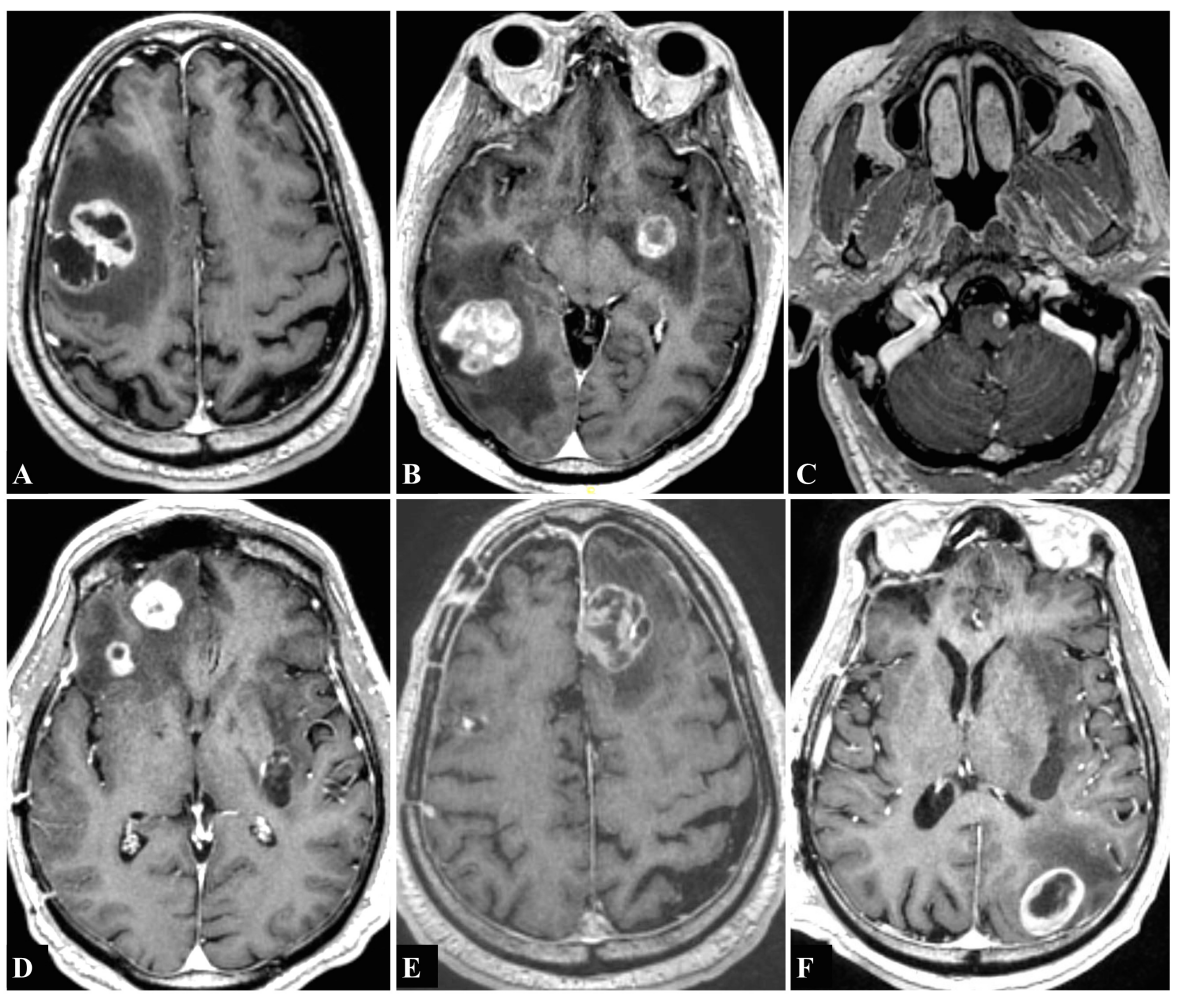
T1-weighted magnetic resonance imaging (MRI) with contrast showing contrast-enhancing lesions Lesions seen in the (A) right middle frontal gyrus (3.5 cm in diameter), (B) left basal ganglia (1.85 cm) and right posterior temporal lobe (4 cm), (C) left medulla (<1 cm), (D) right orbitofrontal cortex (2 cm and 1.25 cm), (E) left parasagittal frontal cortex (2.45 cm), and (F) left parietal cortex (2.4 cm).
